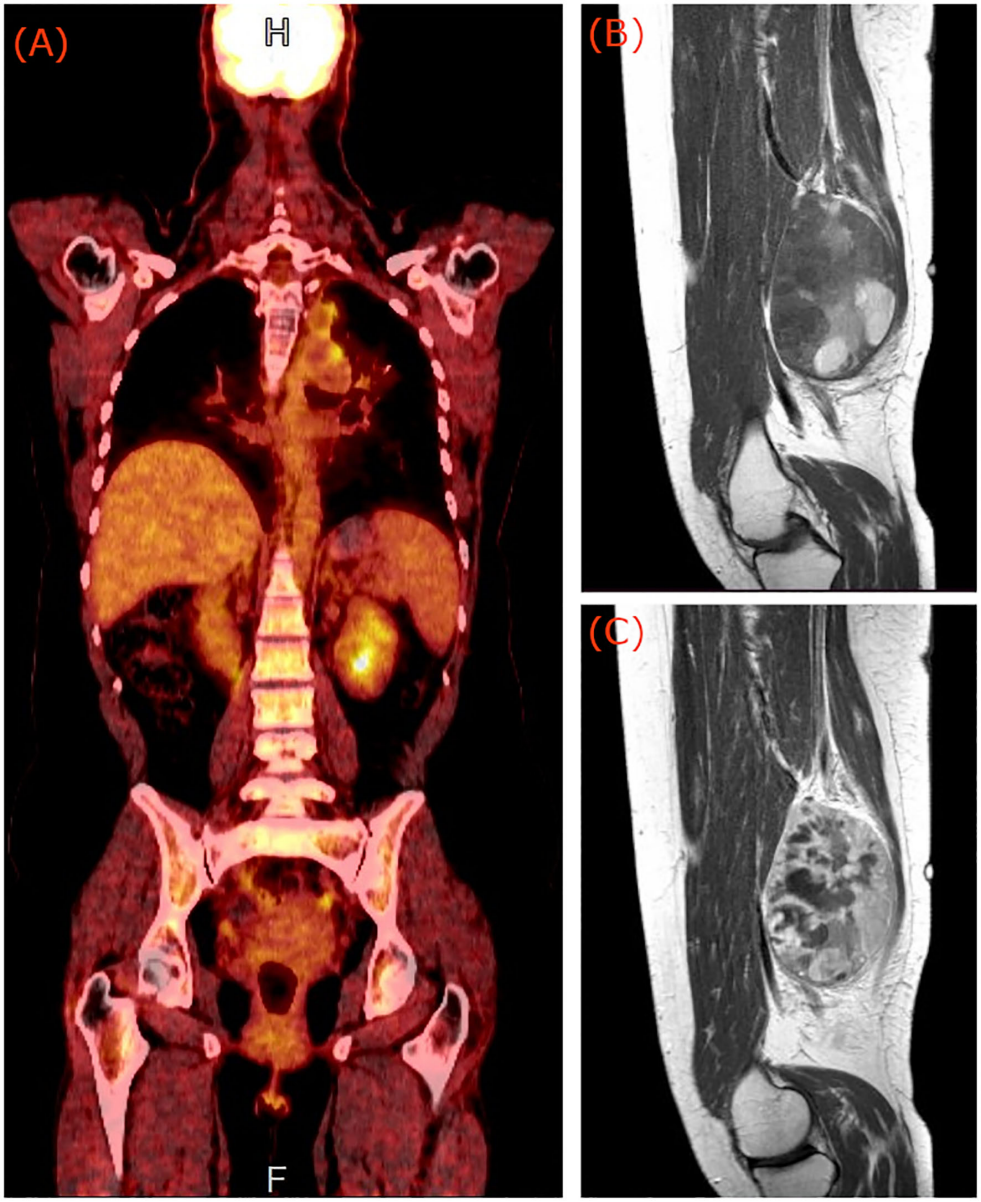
(A) PET/CT of the patient showing medium FDG uptake of the left hilar and mediastinum nodes. Pre (B) and post (C) contrast sagittal T1 weighted magnetic resonance images of the right thigh of the patient, showing a deep-seated lobulated mass, with cystic regions, bleeding elements and strong heterogeneous enhancement indicative of synovial sarcoma.
